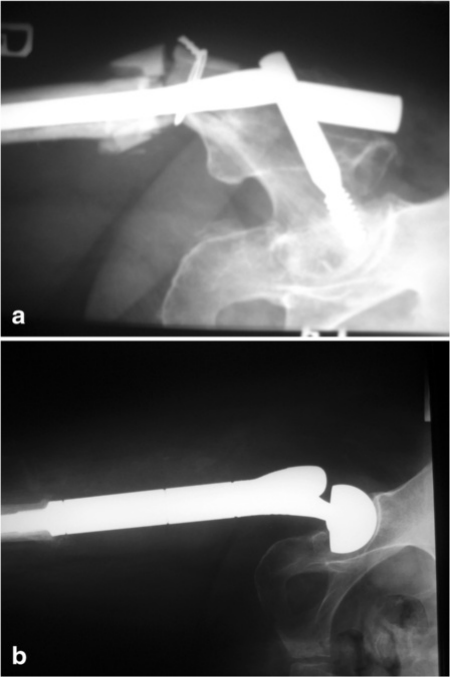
Failed fixation (gamma nail with PMMA) of a proximal femoral fracture due to breast cancer metastasis (a) and radiographs after modular endoprosthesis replacement (b)
